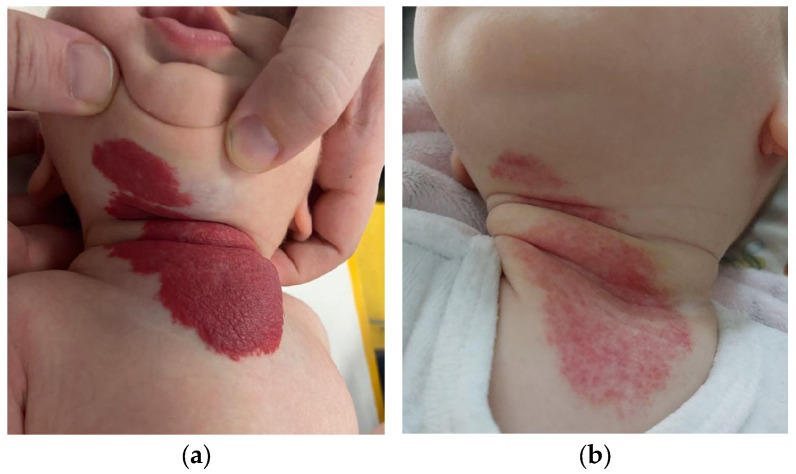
A 12-week-old female infant presented with a mixed IH extending to the neck, chin, and sternal region, requiring multidisciplinary management for treatment with propranolol, including ultrasound and ENT consultation with fiber optic laryngoscopy (a). Telangiectatic residuals were observed after three months of treatment with propranolol (b).
